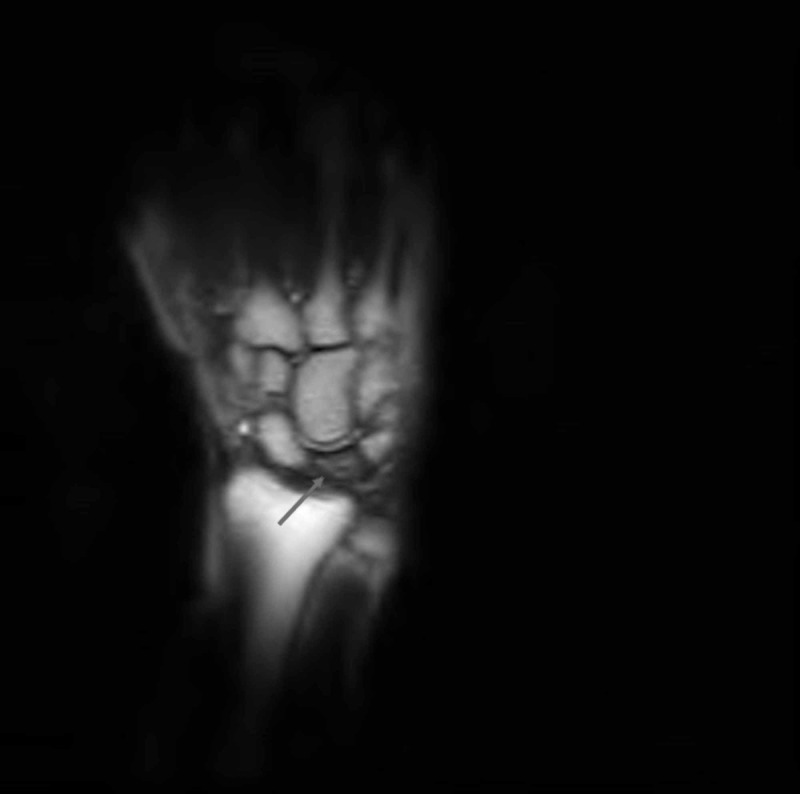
Coronal T1-weighted MR image of the left wrist revealed mild flattening of the lunate, a linear fissure fracture superiorly, and a decreased signal intensity of the lunate suggestive of Kienböck’s disease, stage IIIA.
